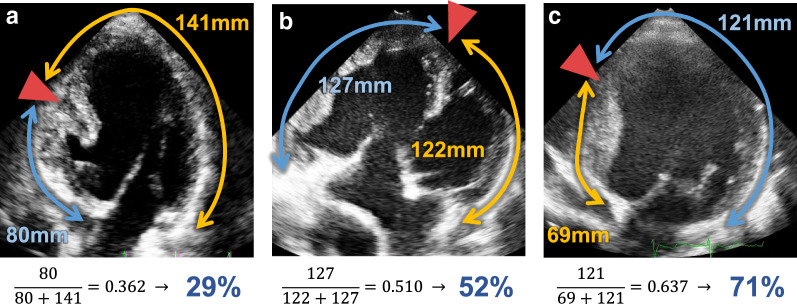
Strategy for estimating the ratio of the delayed wall. From apical four chamber-like views in patients with single ventricle, an insertion point of the septum (red triangles), which separates a ventricular wall into two regions, can be detected. Representative cases of the ventricle with large ventricular septal defect and a hypoplastic chamber, the codominant-type ventricle, and the ventricle with intact ventricular septum and a hypoplastic chamber of closed cavity outside of the systemic ventricle are shown in Panels a, b, and c, respectively. Using the two-dimensional speckle tracking echocardiography, we can judge a wall region that exhibits a relatively delayed contraction by comparing the times to peak strain between the two regions. From longitudinal lengths of the earlier activated and delayed ventricular wall regions (yellow and blue lines, respectively), the length ratio of the delayed region is calculated (formulas shown at the bottom left of each panel). The length ratio is converted into the area ratio (blue percentages shown at the bottom right of each panels) (see Additional file 1: Appendix A)
